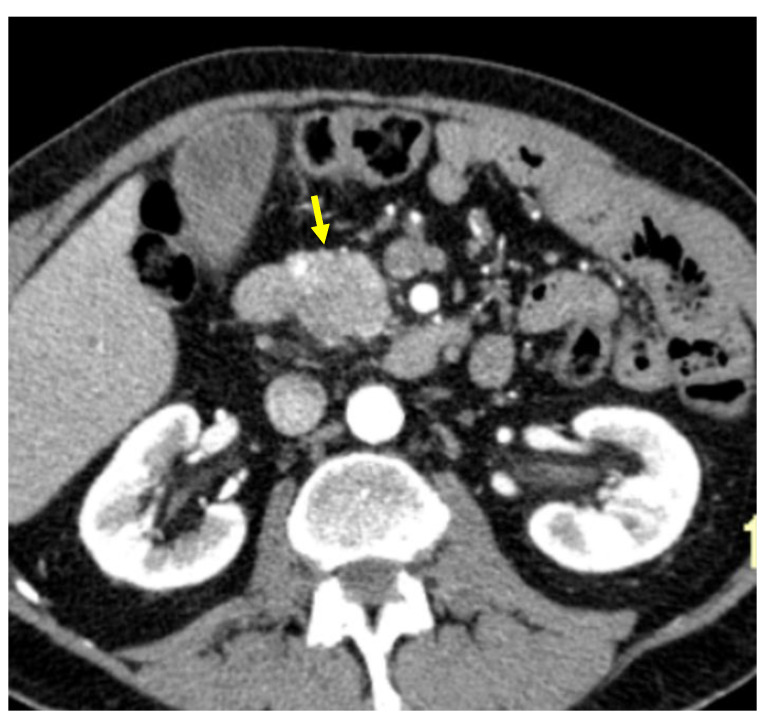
Pancreatic lesion with well-defined margins (arrow) on CT examination in pancreatic contrast phase.
